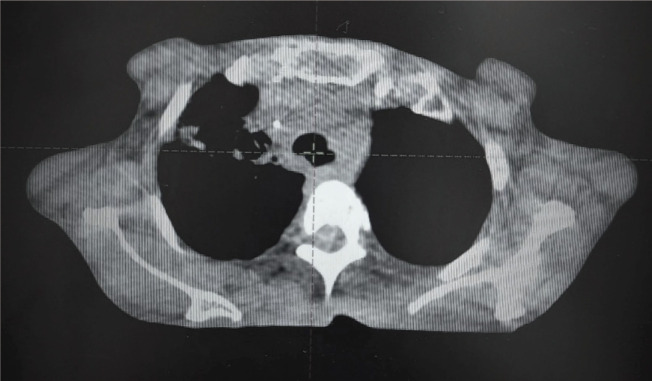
CBCT at last RT fraction showing complete resolution of tracheal compression.
